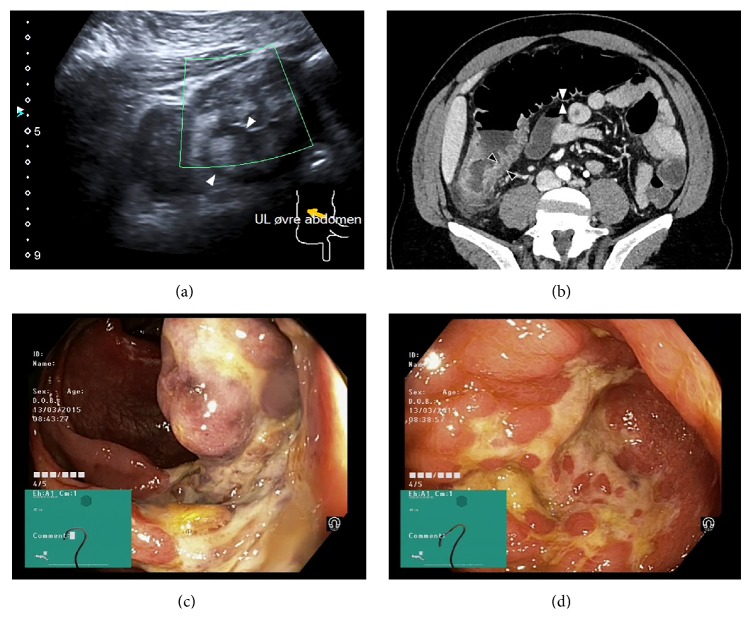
(a) Transabdominal ultrasound. Cecal lumen is compromised by the thickened bowel wall. (b) Computed tomography scan of the right colon. Ascending colon is thickened with intramural edema. (c) Endoscopic image from ascending colon. Image shows ischemic transformation with edema and ulcerations. (d) Endoscopic image from cecum. Circumferential alterations with fibrin-covered ulcerations.
